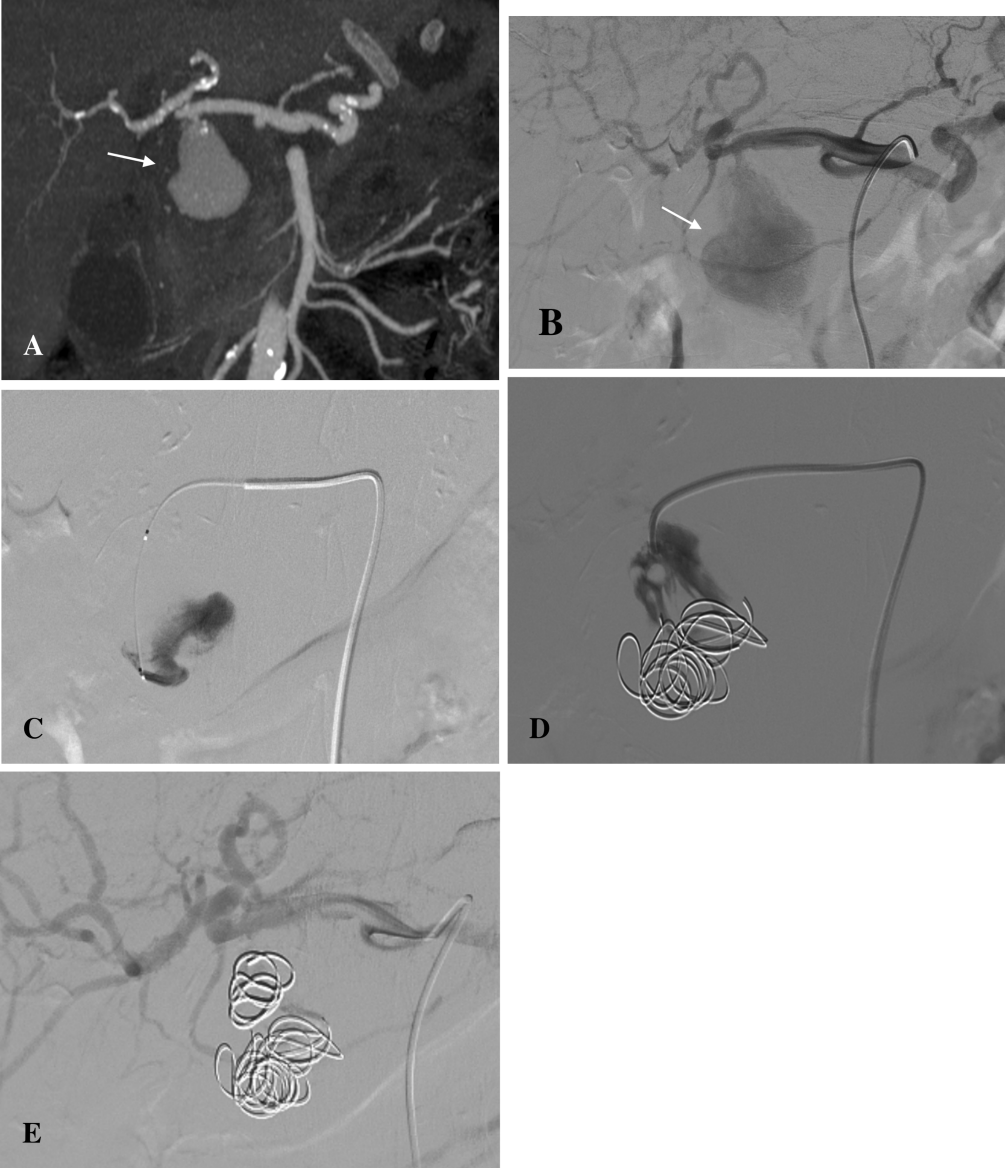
Pseudoaneursym of the common hepatic artery treated with coil and thrombin embolization. (A) Contrast-enhanced CT scan and (B) DSA image showing the pseudoaneursym arising from the distal common hepatic artery (arrow). (C) DSA image showing initial thrombin injection. (D) Persistent perfusion despite additional placement of coils. (E) Final DSA depicting procedural success after the deployment of an additional coil and thrombin.
